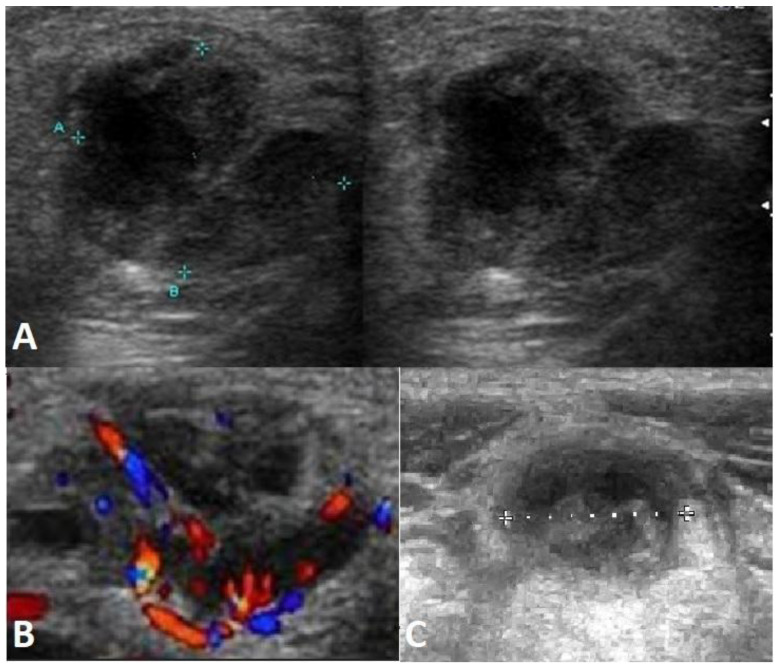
Ultrasound scans of pathological lymph nodes. (A) Lymph nodes with the potential breakup. (“A”, “B” indicate the borders of pathological lymph nodes) (B) Lymph nodes with incorrect vascular flow. (C) Inhomogeneous lymph nodes.
